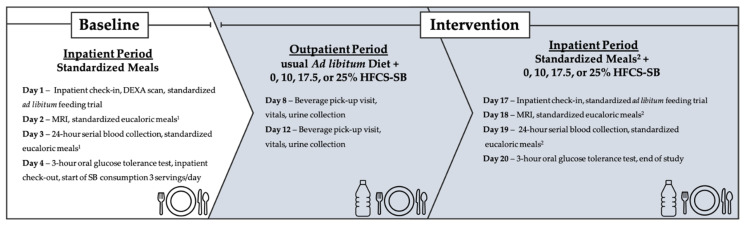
Study design and experimental testing and collection days. DEXA, dual energy X-ray absorptiometry; MRI, magnetic resonance imaging; SB, sweetened beverage; HFCS, high fructose corn syrup. ^1^ Fifty-five percent complex carbohydrate (<2%added sugar), 35% fat, 15% protein. ^2^ 55–30% complex carbohydrate (depending on assigned SB, <2%added sugar), 35% fat, 15% protein. % = % of energy requirement.
